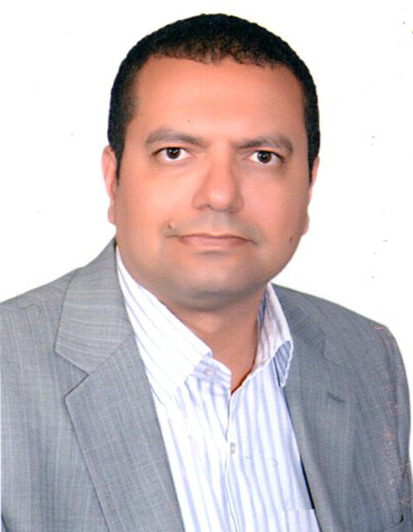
Dr Hesham Naeim, MD, FASE graduated from Faculty of Medicine, Al-Azhar University at December 1997. Granted M.Sc. degree in cardiovascular diseases at December 2002. Granted MD degree in cardiovascular diseases and interventions 2006. Diplomate - Adult Comprehensive Echocardiography from National Board of Echocardiography, United States at June 2014. Resident and Assistant Lecturer of Cardiology, in Al-Azhar University hospitals from June 1997 to February 2006. Cardiology consultant in MNH Saudi Arabia from January 2007 till April 2013. Adult cardiology consultant in Madinah Cardiac Center Saudi Arabia from June 2013 till now. Dr Hesham is expert in Echocardiography in structural heart disease.
